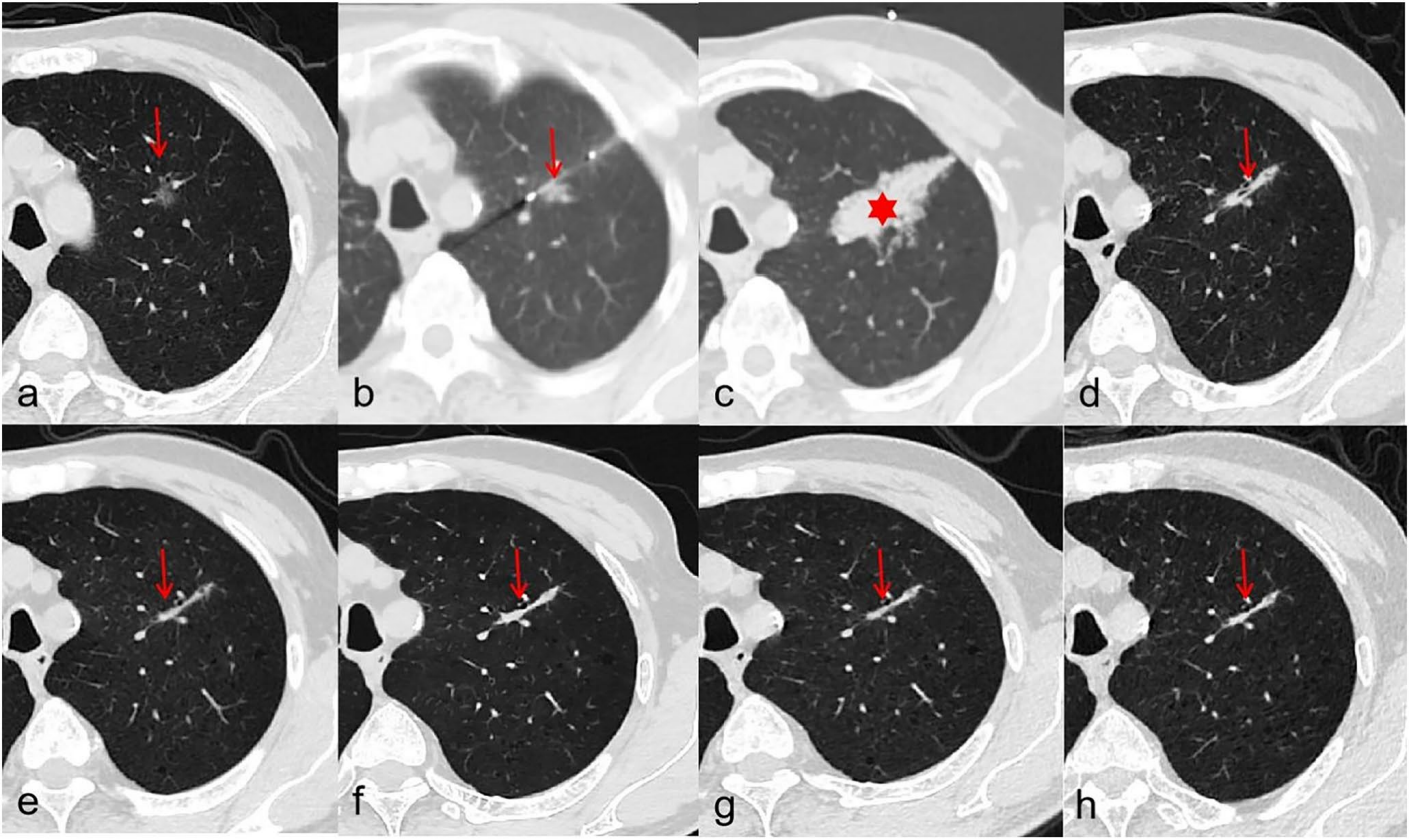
A 73‐year‐old male with a sub‐solid nodule presented a percutaneous cryoablation in the left upper lobe. (a) Before the operation, he accepted a HRCT examination to locate the lesion (arrow). (b) CT scan showing a coaxial introducer needle (arrow) which was placed abutting the lesion. (c) In the CT scan immediately after cryoablation, the ablated nodule presented an “ice ball”—a circular ground‐glass opacity (star). (d–h) The follow‐up CT scans performed 1, 3, 6, 12 and 24 months, respectively, after cryoablation. The lesion didn't recur, which showed a significantly effective result.
